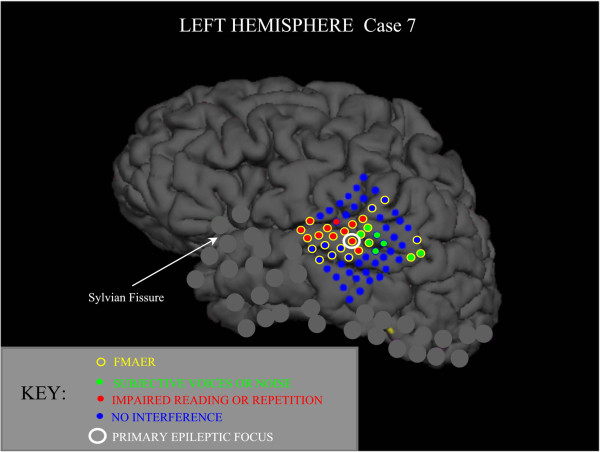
Cortically recorded FMAER; high electrode density grid, one subject. The true lateral surface and grid contact locations are shown as reconstructed from MRI and CT studies. The superior surface (vertex) is shown above, inferior temporal surface below, prefrontal lobe to left and occipital pole to right. An 8 × 8 (64 contact) high density grid is shown as placed over the left temporal region where contact centers are 0.5 cm apart. The FMAER was recorded from electrodes surrounded by yellow circles. Red dots signify contacts exhibiting impairment of reading and/or of speech repetition during cortical stimulation. The green dots are regions where the patient reported hearing voices or noise. The single contact showing the primary epileptic focus is surrounded by a larger white circle. Blue dots represent contacts not found to interfere with language function during cortical stimulation. Contacts of additional standard grids placed elsewhere are grayed out to avoid image clutter. None of these grayed out standard grid contacts demonstrated FMAER responses.
